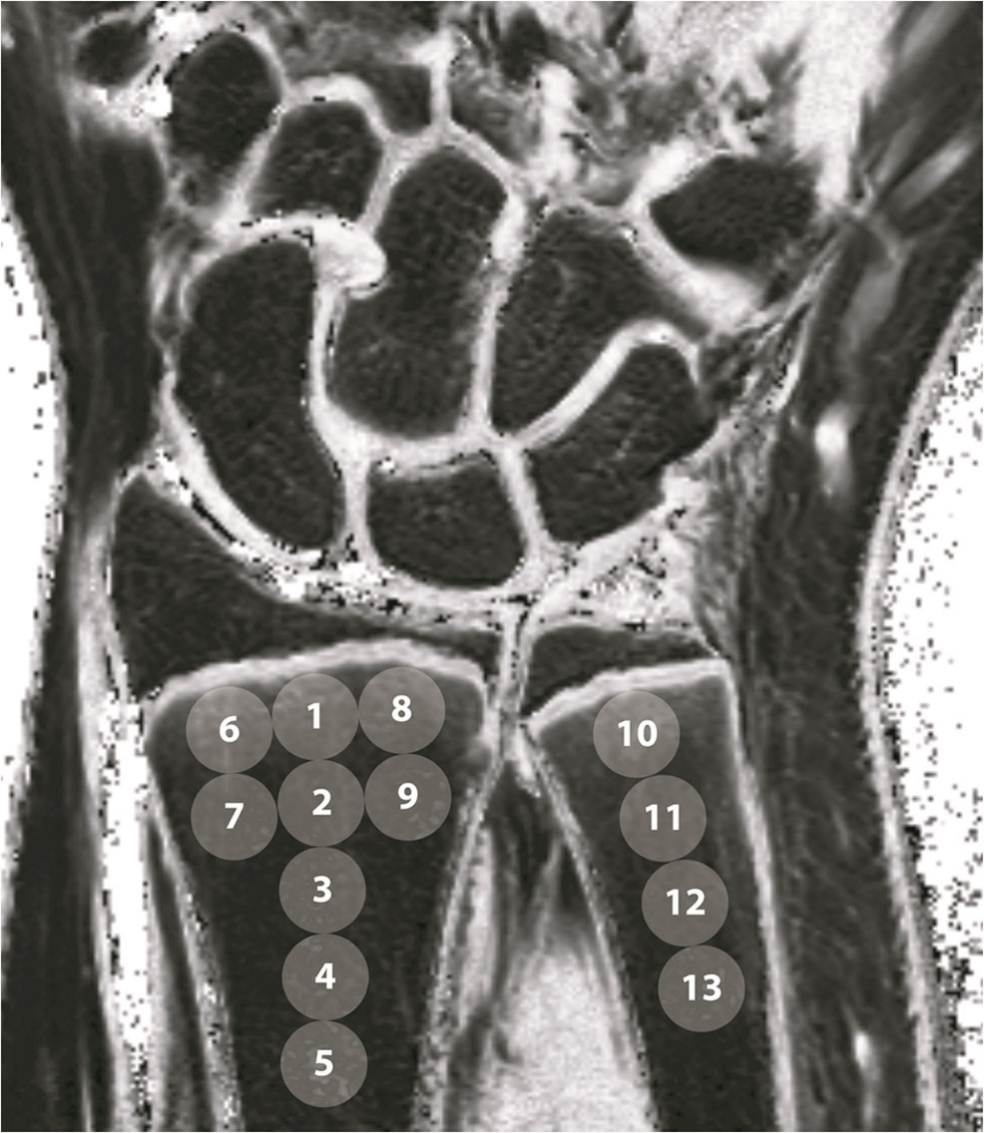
Placement of regions of interest on post-processed coronal T1-weighted and T2-weighted Dixon images. ROIs 1, 6, 8, and 10 were placed directly adjacent to the physis, representing most distal 0–5 mm of the metaphysis
